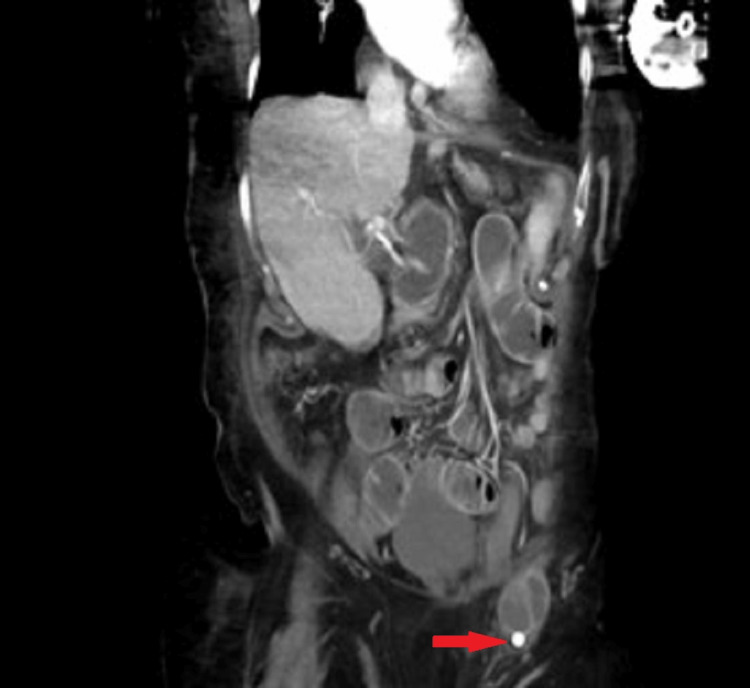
CT image shows a radiopaque shadow in the hernia sac, which proved to be a gallstone (arrow).
